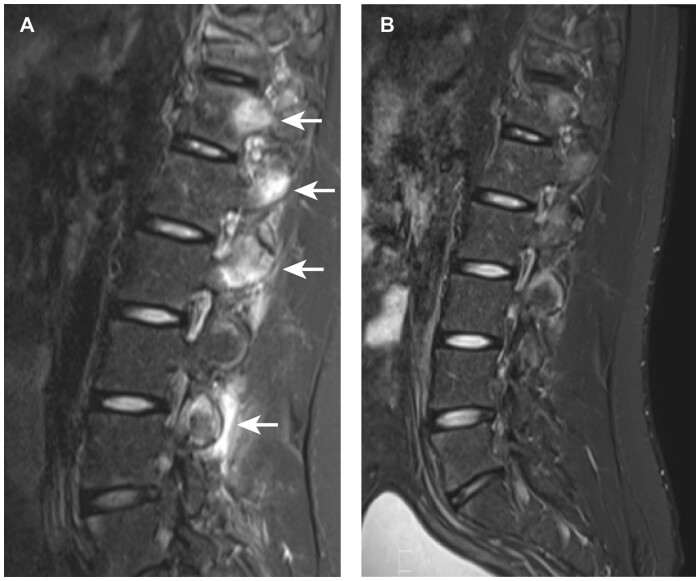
Short tau inversion recovery MRI at (A) baseline and (B) week 12 from a patient who had received filgotinib. MRI scans demonstrate posterolateral inflammation (top arrow), facet joint inflammation (middle two arrows) and soft-tissue inflammation (bottom arrow) in the lumbar spine that resolved after treatment
